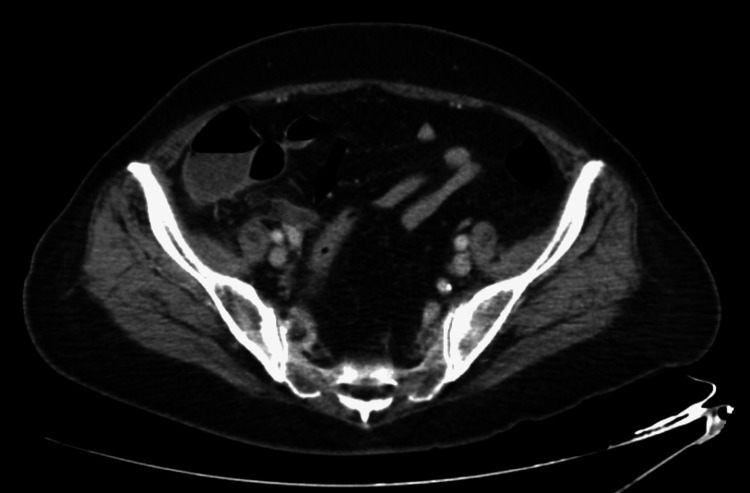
Axial enhanced computed tomography scan showing a distended and fluid-filled distal appendix (black arrow) with wall thickening, as well as surrounding fat stranding.
